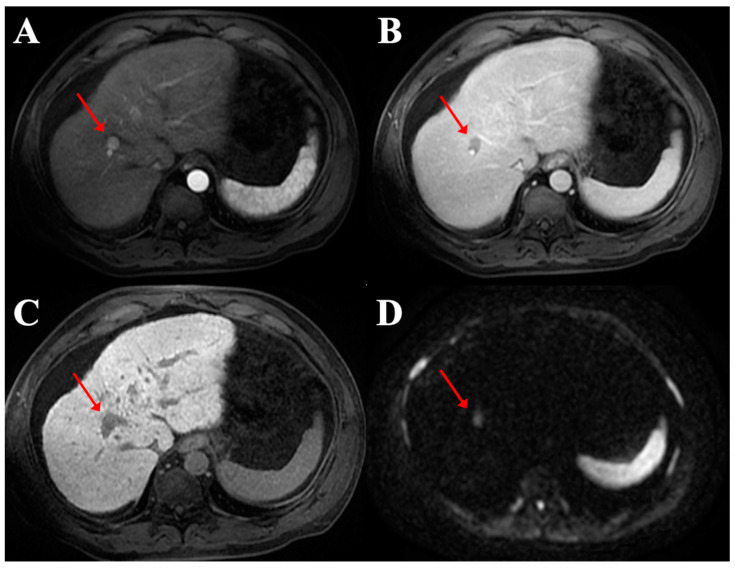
Magnetic resonance imaging of the axial planes of a HCC nodule (red arrows) in segment 8 showing hyperenhancement in the arterial phase (A), wash-out in the portal phase (B), hypointensity in the hepato-biliary phase (C) and diffusion restriction on the diffusion weighted imaging (DWI) using b-values of 800 s/mm^2^ (D).
